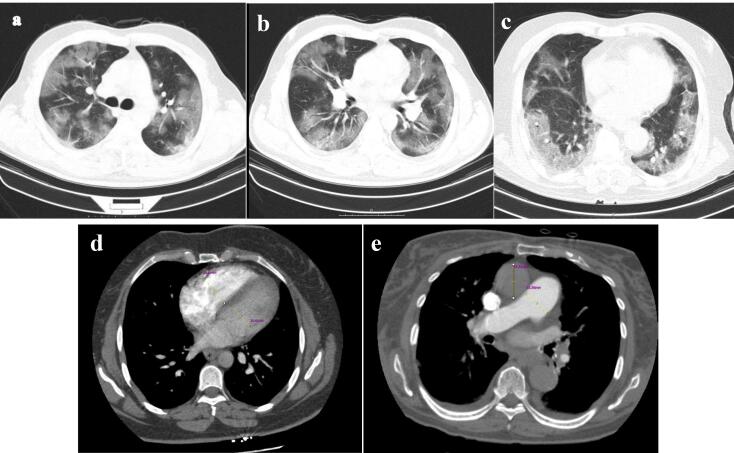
###  Chest CT severity score
